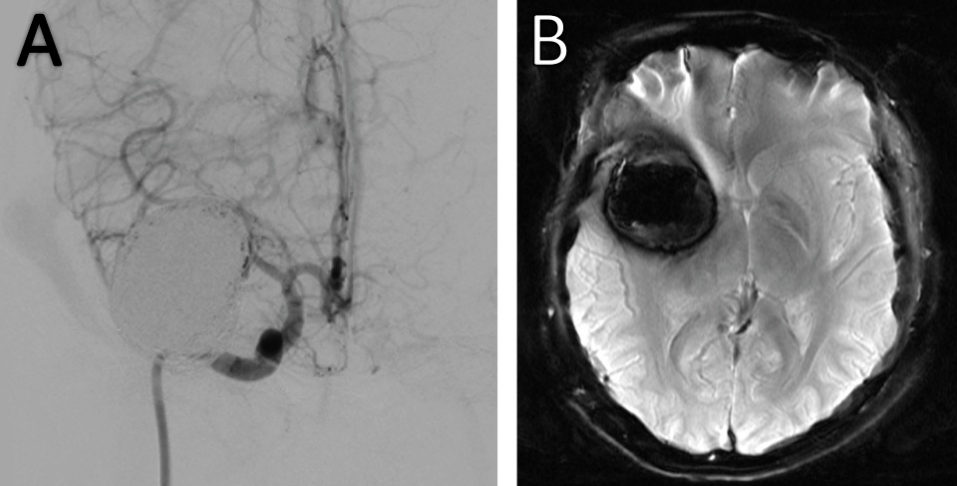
Post-embolization MRI and Catheter Angiography Images (A) Catheter angiography and (B) Susceptibility-Weighted Magnetic Resonance Imaging post-coiling one-year-control demonstrating adequate embolization with thrombosis and without partial filling of the aneurysm.
